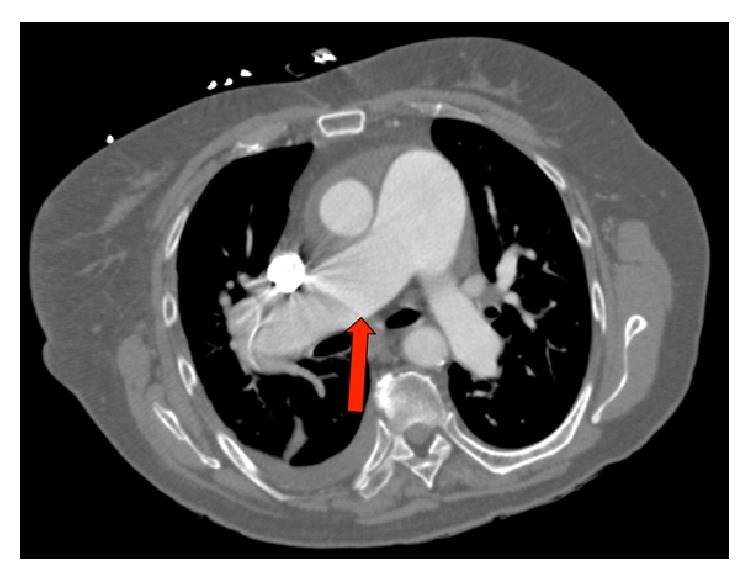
CT-scan showing enlarged pulmonary artery (red arrow).
